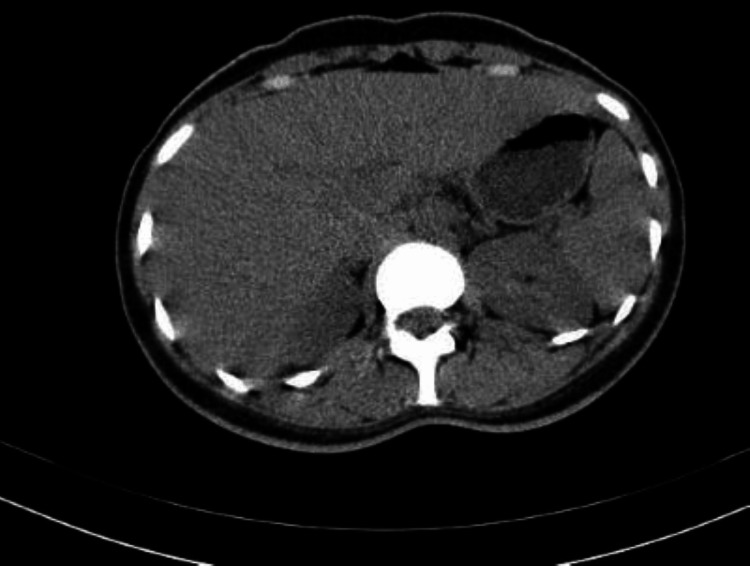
Computed tomography (CT) of the abdomen showing the liver and no evidence of biliary obstruction.
